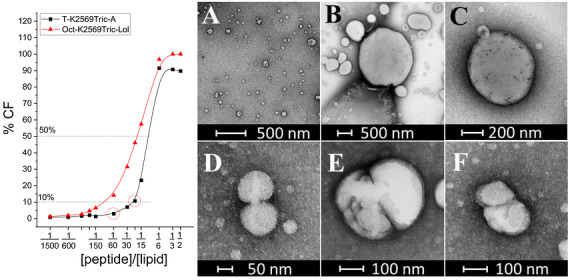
The circles highlight the percentages of CF leakage obtained at P/L 1:20 (10% leakage, corresponding to TEM panels B‐C) and 1:60 (leakage below 5%, corresponding to TEM panels D‐F) for T‐K2569Tric‐A. TEM images recorded for the pristine SUVs are reported in panel A.
